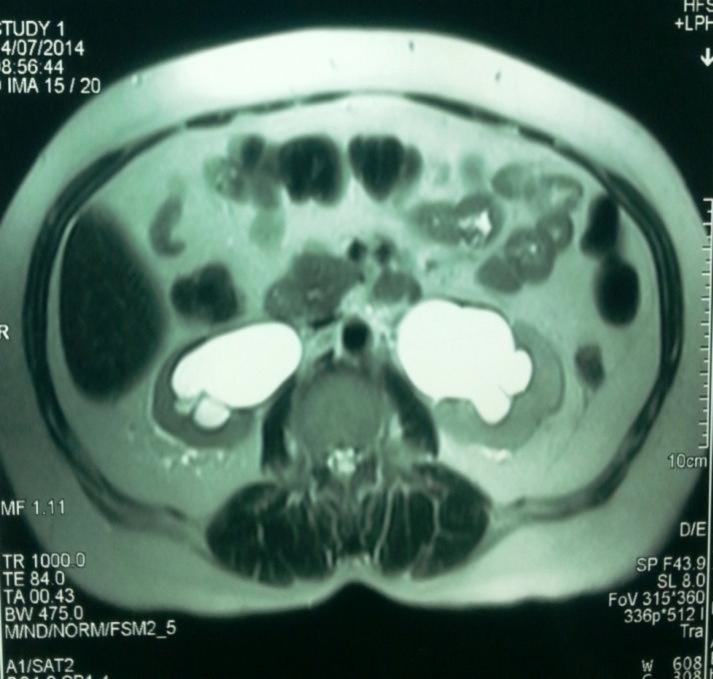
50-year-old female patient with invasive lobular breast carcinoma metastatic to the urinary bladder. Contrast Enhanced MRI image of the pelvis, axial reconstruction demonstrating: bilateral hydronephrosis
